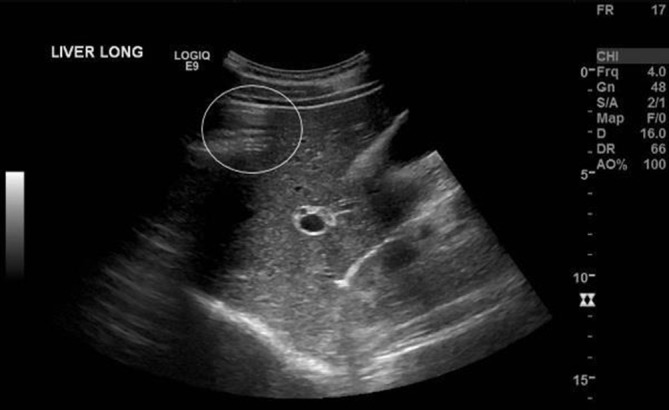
Sagittal image of the liver through segments 8 and 5. The peritoneal stripe sign can be appreciated obscuring the superior aspect of segment 8. There was no appearance of gallstones and Murphy’s sign was negative on ultrasound probe pressure.
